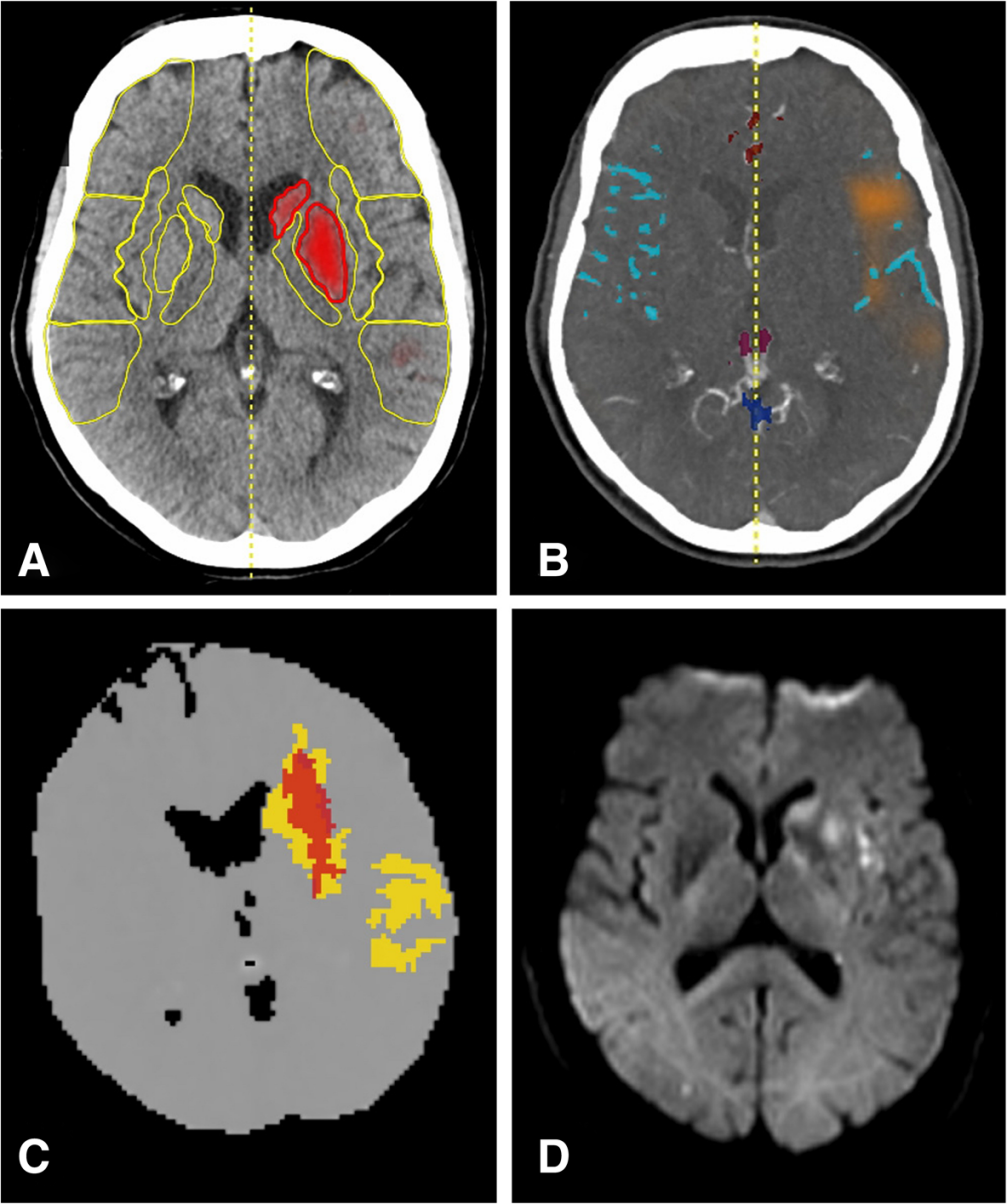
Acute LVO with sufficient collateral flow, successful recanalization and good outcome: A 77-year-old woman suffered from an acute hemiparesis and aphasia (NIHSS 17) due to an left-sided M1 occlusion. As in case 1, e-ASPECTS (a) was 8 with the caudate head and lentiform nucleus being affected, but e-CTA collateral score was 2 (54%, b), and the hypoperfused area (c) is considerably smaller. Again, full recanalization (mTICI 3) could be achieved within 5 h from symptom onset, and the patient recovered completely (NIHSS 0). Follow up MRI at 36 h (d) shows incomplete infarction of the striate only
